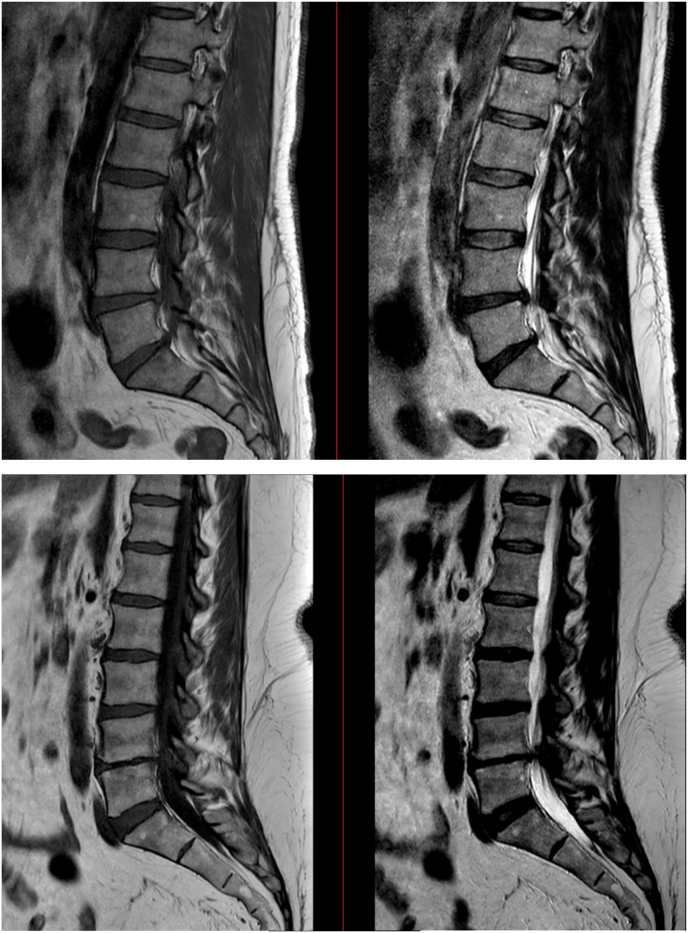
Disc herniation at L4-5. Upper panel: Sagittal T1-and T2-weighted MRI images showing normal bone marrow intensity and absence of Modic changes. Lower panel: Sagittal T1-and T2-weighted MRI images showing hyperintense areas around the L4-5 disc, indicating type II Modic changes.
